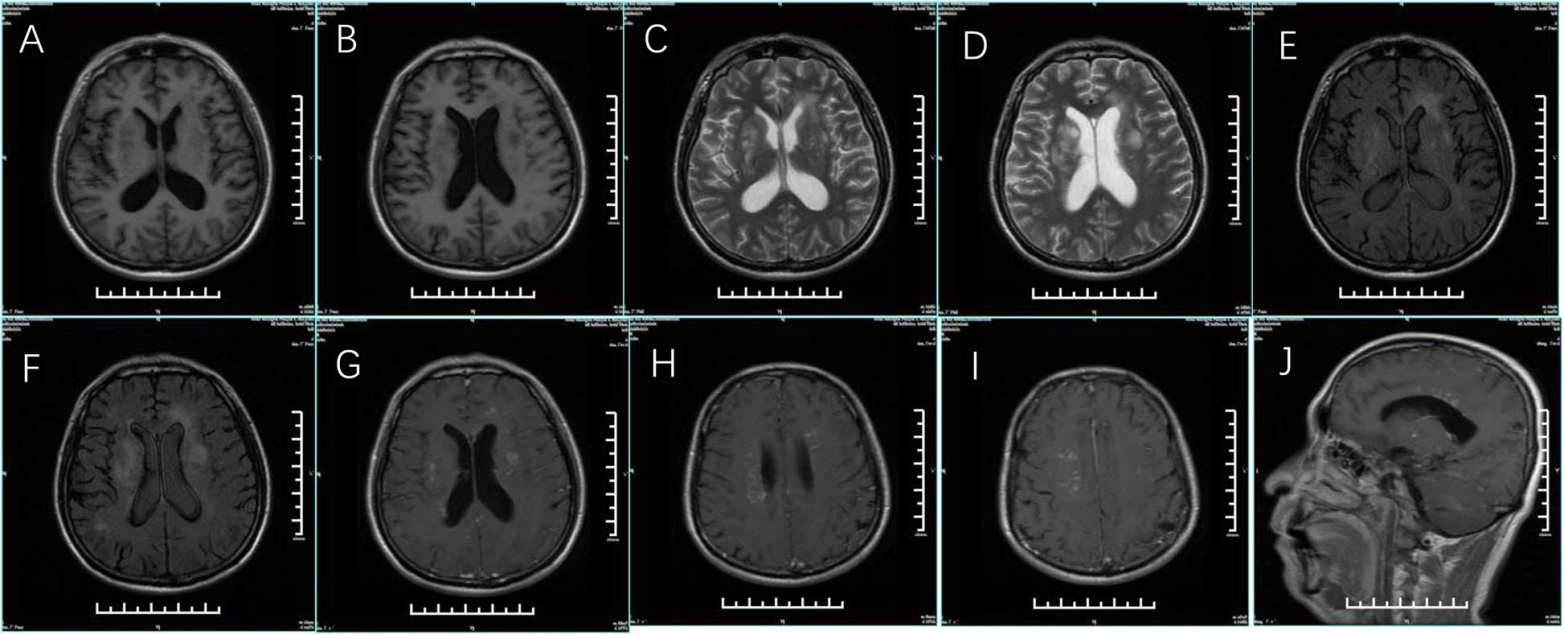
Magnetic resonance imaging of the patient. Magnetic resonance imaging of the patient. T1 weighted image revealed a low signal in the lesion area (a-b). T2-weighted fluid-attenuated inversion recovery (FLAIR) showed elevated signals within bilateral paraventricular, radiate corona, semioval center, and right subcortex (c-f), contrast-enhanced scans showed patchy and linear perivascular radial gadolinium enhancement in the above areas (g-j)
